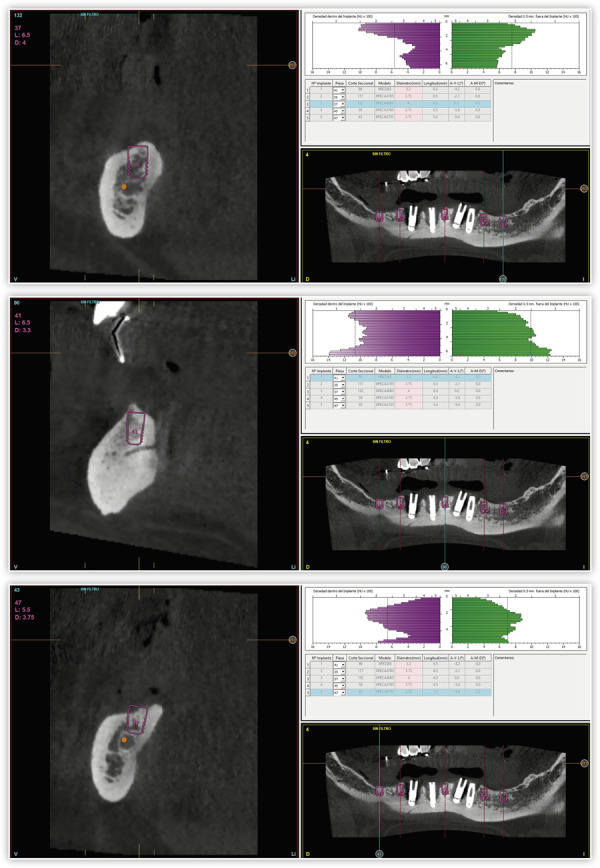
Imágenes del cone-beam de planificación.
